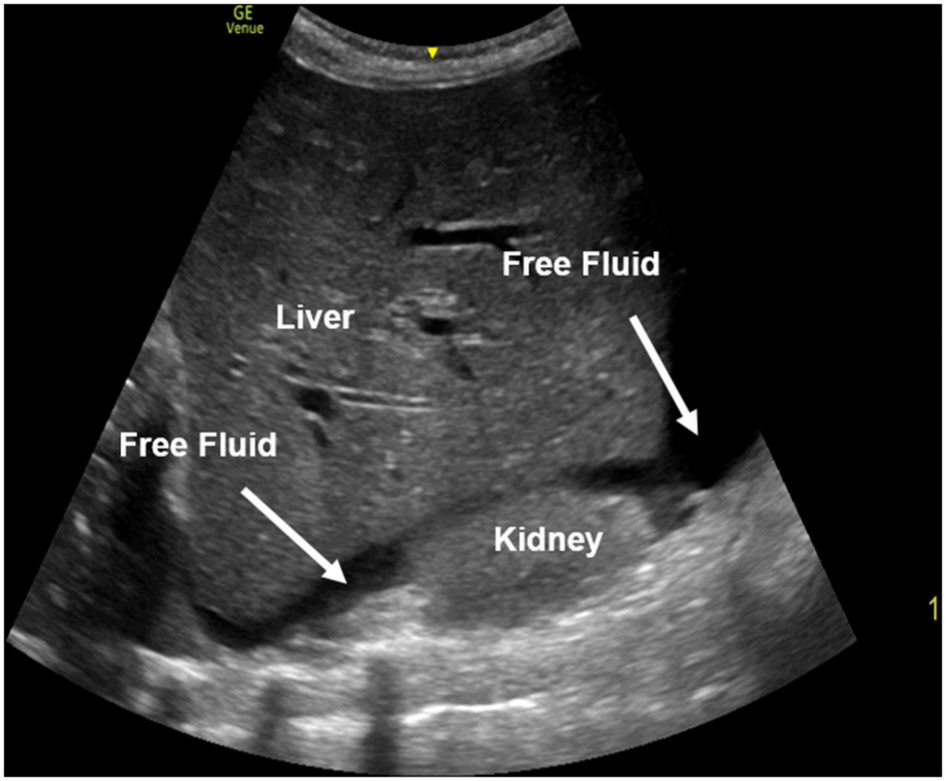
A right upper quadrant view performed in a FAST exam with free fluid present between the liver and the kidney. The free fluid appears black (anechoic) on ultrasound.
